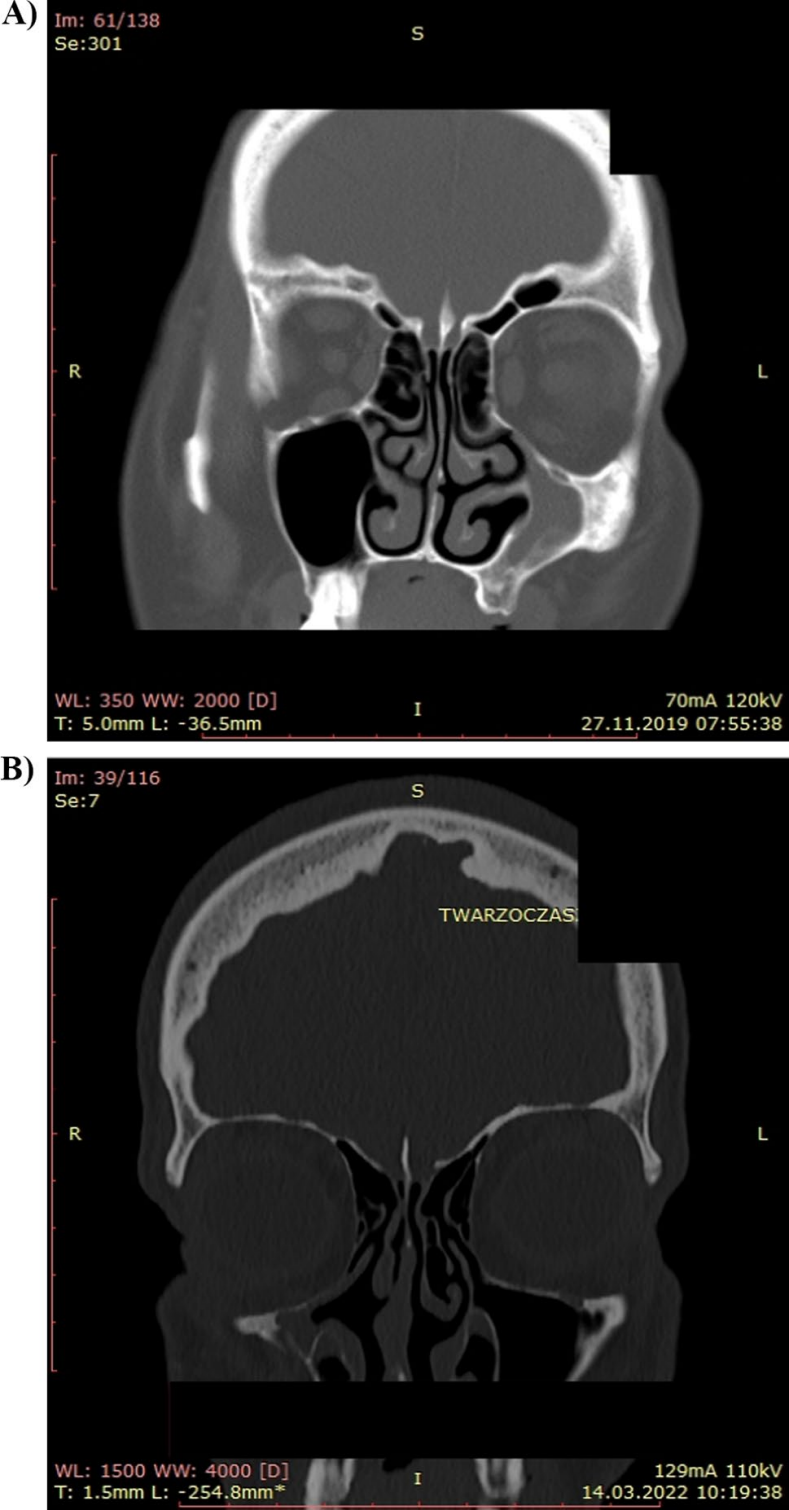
CT images, A–B show the silent sinus syndrome involving the maxillary sinus. A 58-year-old female with SSS of the left maxillary sinus. B 40-year-old female with SSS of the right maxillary sinus
